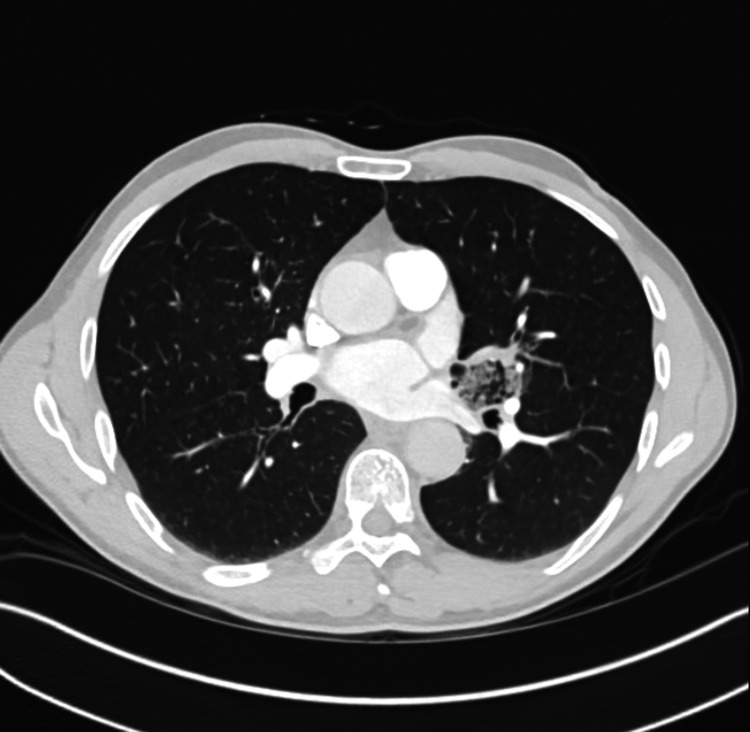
Computed tomography scan showing a left perihilar cystic lesion near the lingula.
